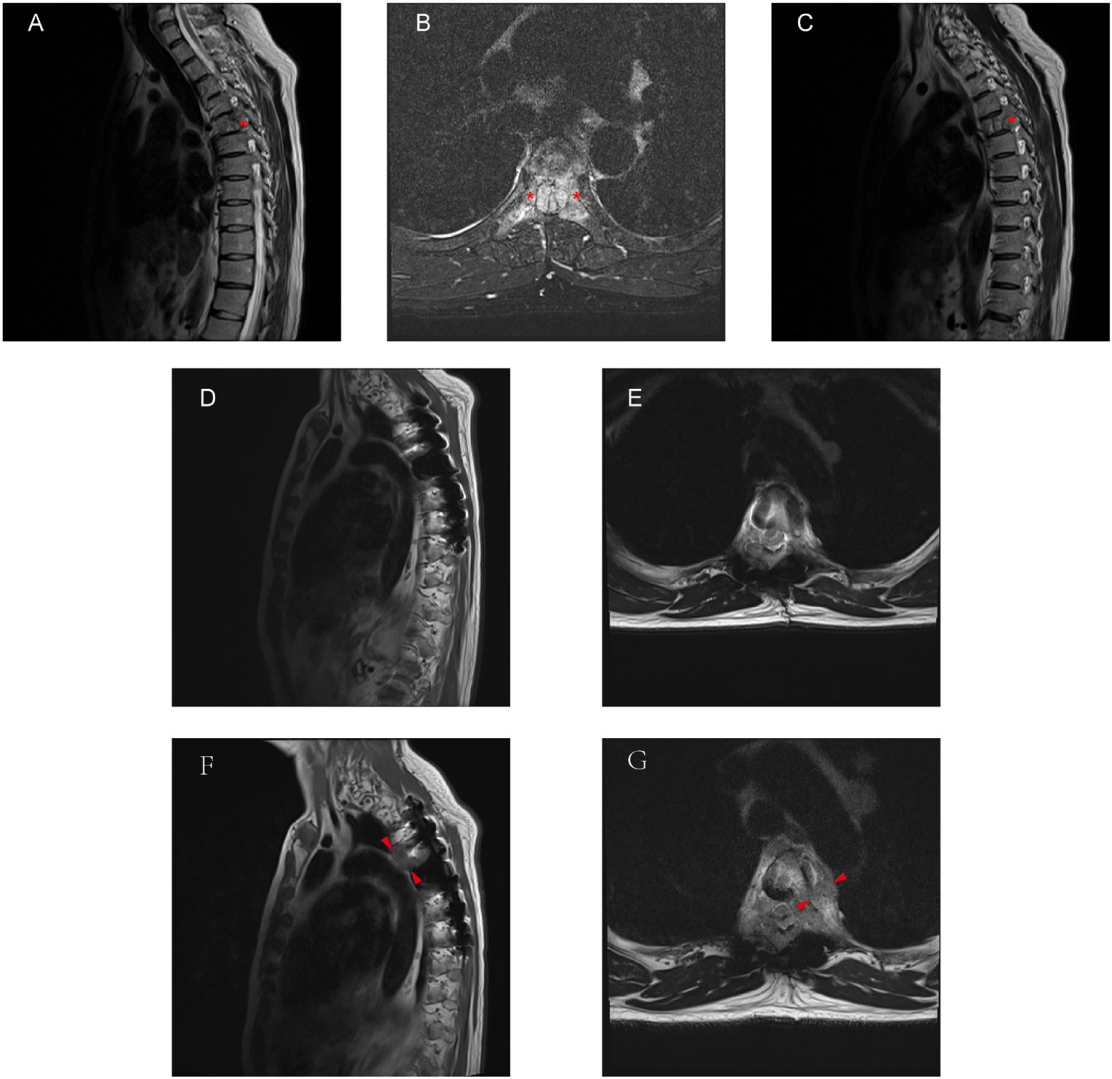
A 47-year-old man with T5 metastases from parotid acinic cell carcinoma A-C. Preoperative MRI displayed the lesion in the T5 vertebra, and both pedicles were involved in the sagittal (A, C) and transversal (B) MRI images. D-E. Six months after operation, MRI images showed no clue of the local recurrence. F-G. Thirty-six months later, as showed in the sagittal (F) and transversal (G) MRI images, there was a clear lesion site near the primary surgical region. Asterisks: pedicles with abnormal signal in MRI; Arrowheads: lesion site.
